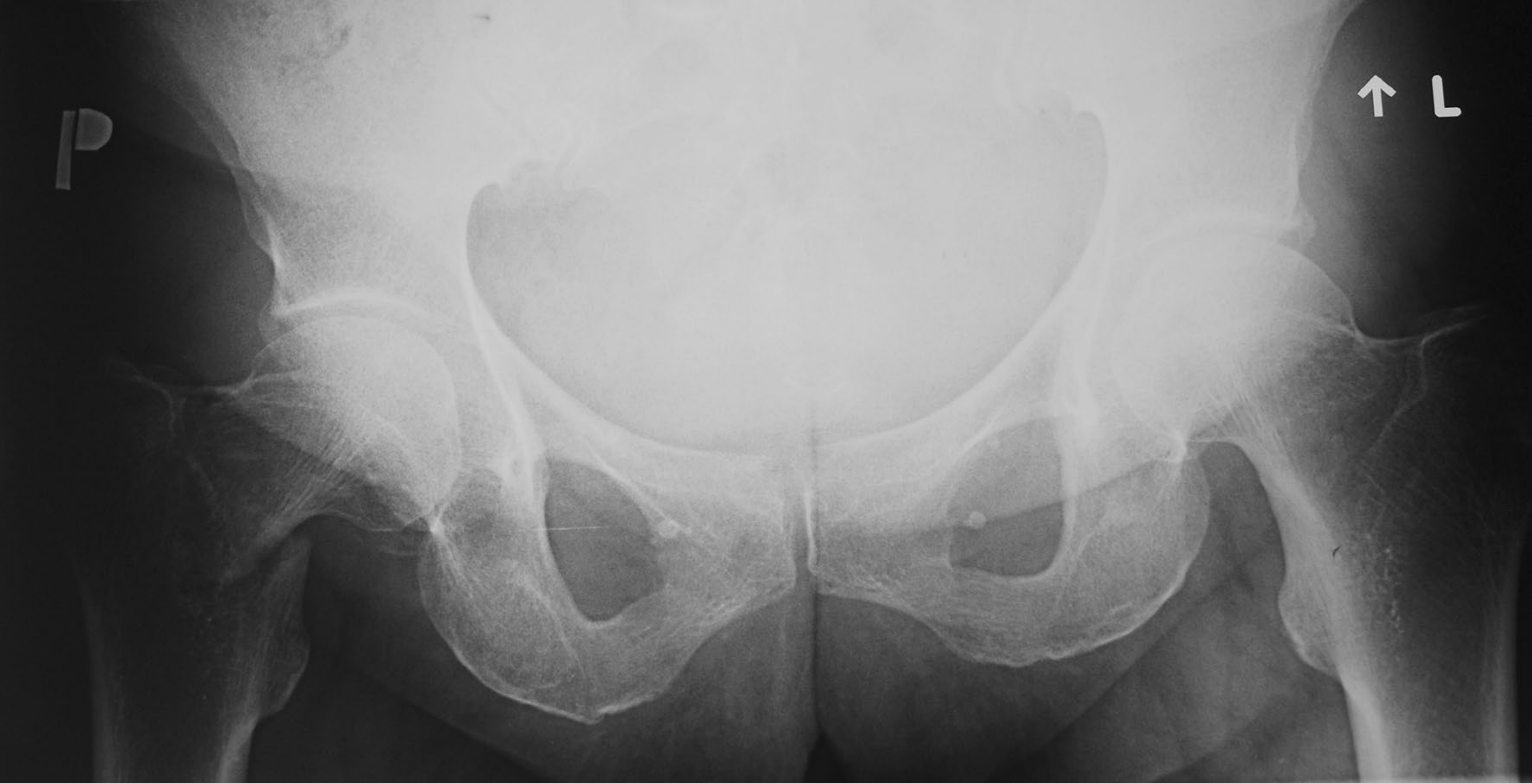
The anteroposterior pelvis radiograph with a basicervical fracture of the proximal right femur
